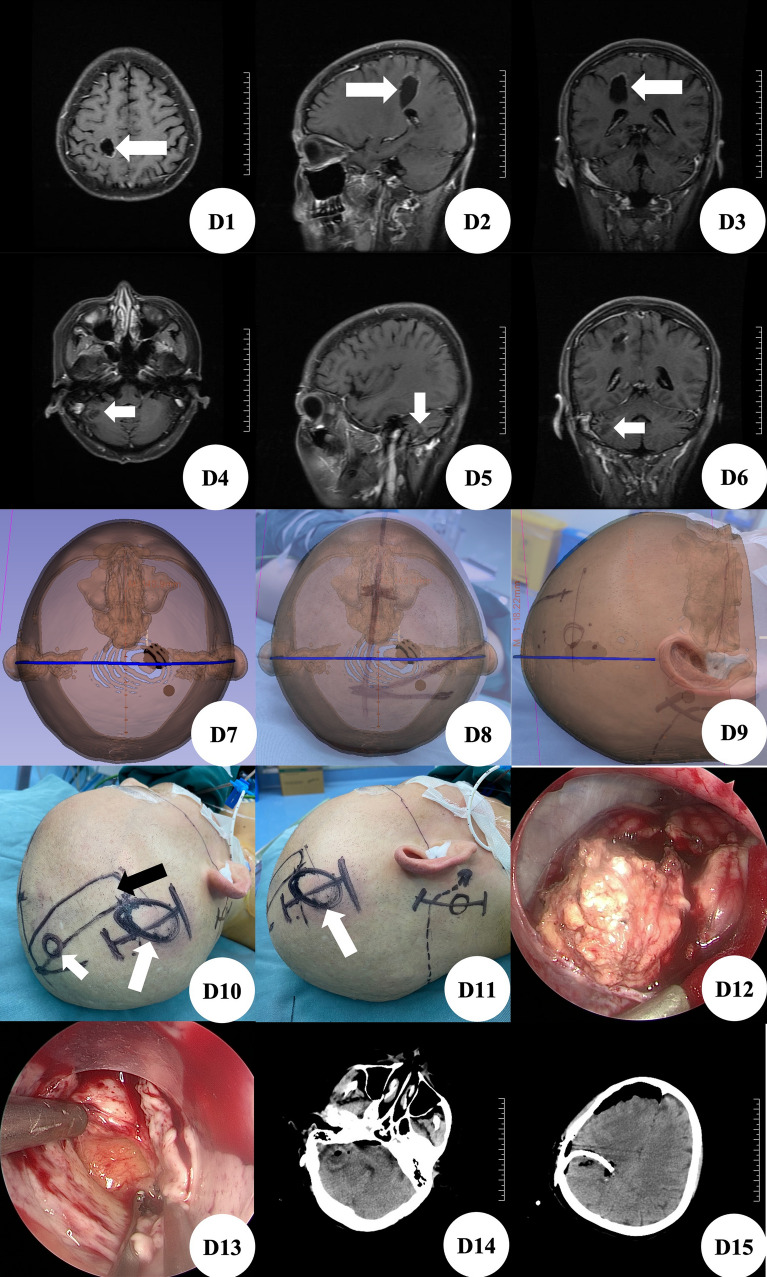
(D1–D3) Brain MRI enhancement showed cystic space occupying lesions in the right frontal parietal lobe, indicated by the white arrow; (D4–D6) brain MRI enhancement showed solid space occupying lesions in the right cerebellum, indicated by the white arrow; (D7) 3D Slicer was used to reconstruct the space occupying image of the right frontal parietal lobe; (D8,D9) use the Apple mobile phone APP MosoCam to show the positive and lateral projection of the right top space occupation; (D10,D11) according to the tumor location located by MosoCam surgical software and the designed surgical incision, the black arrow is the body surface projection of the central anterior gyrus, and the white arrow is the frontal and lateral projection of the right frontoparietal lobe respectively; (D12) intraoperative neuroendoscopic images of right cerebellar mass; (D13) intraoperative neuroendoscopic images of right parietal frontal space occupation; (D14,D15) postoperative brain CT showed that the tumor was resected cleanly without delayed bleeding.
